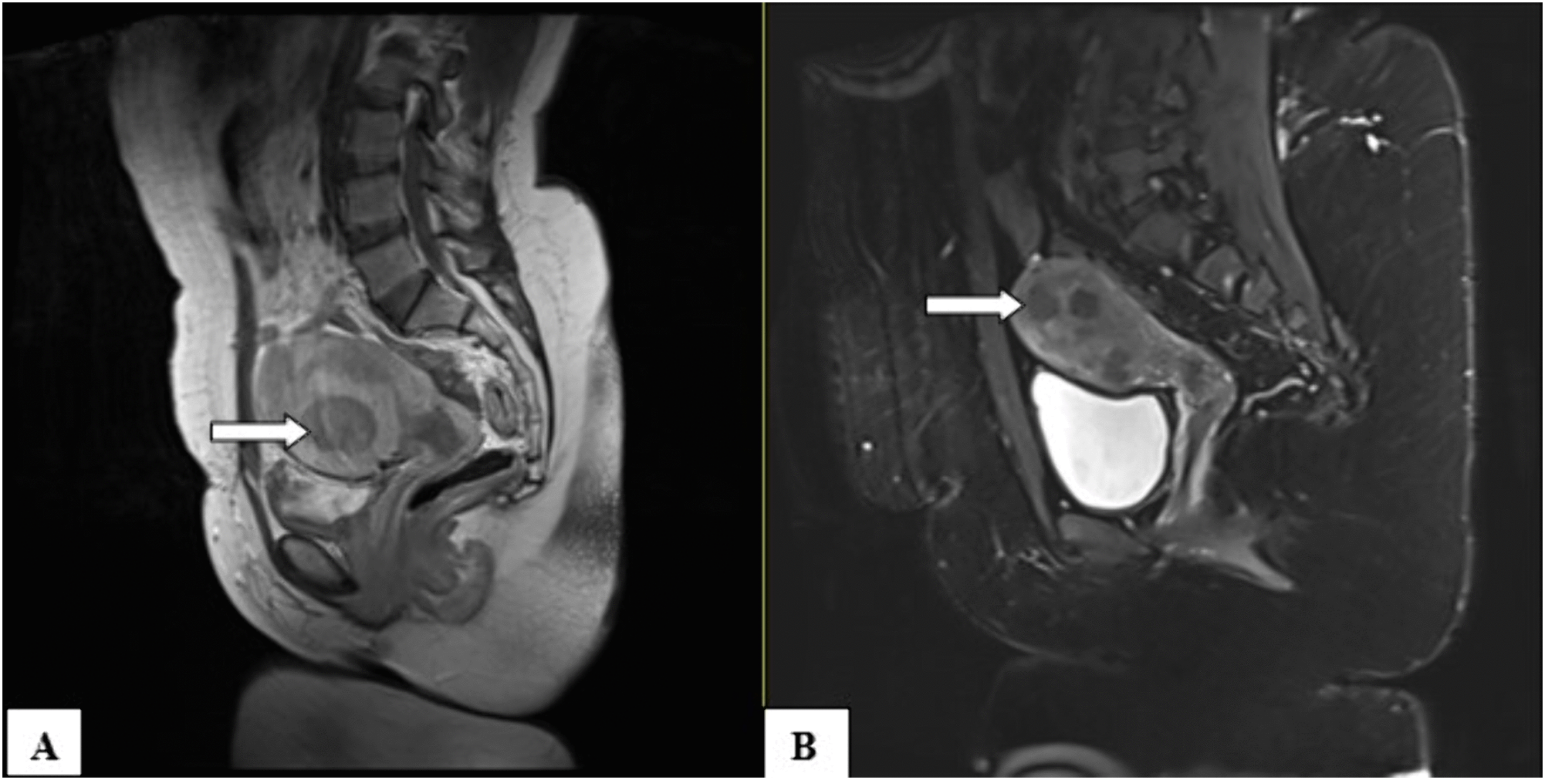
MRI pelvis done at MNH, T2W sequences (Image B with fat suppression) in sagittal plane from 2 different patients aged 35 and 39 years respectively.
